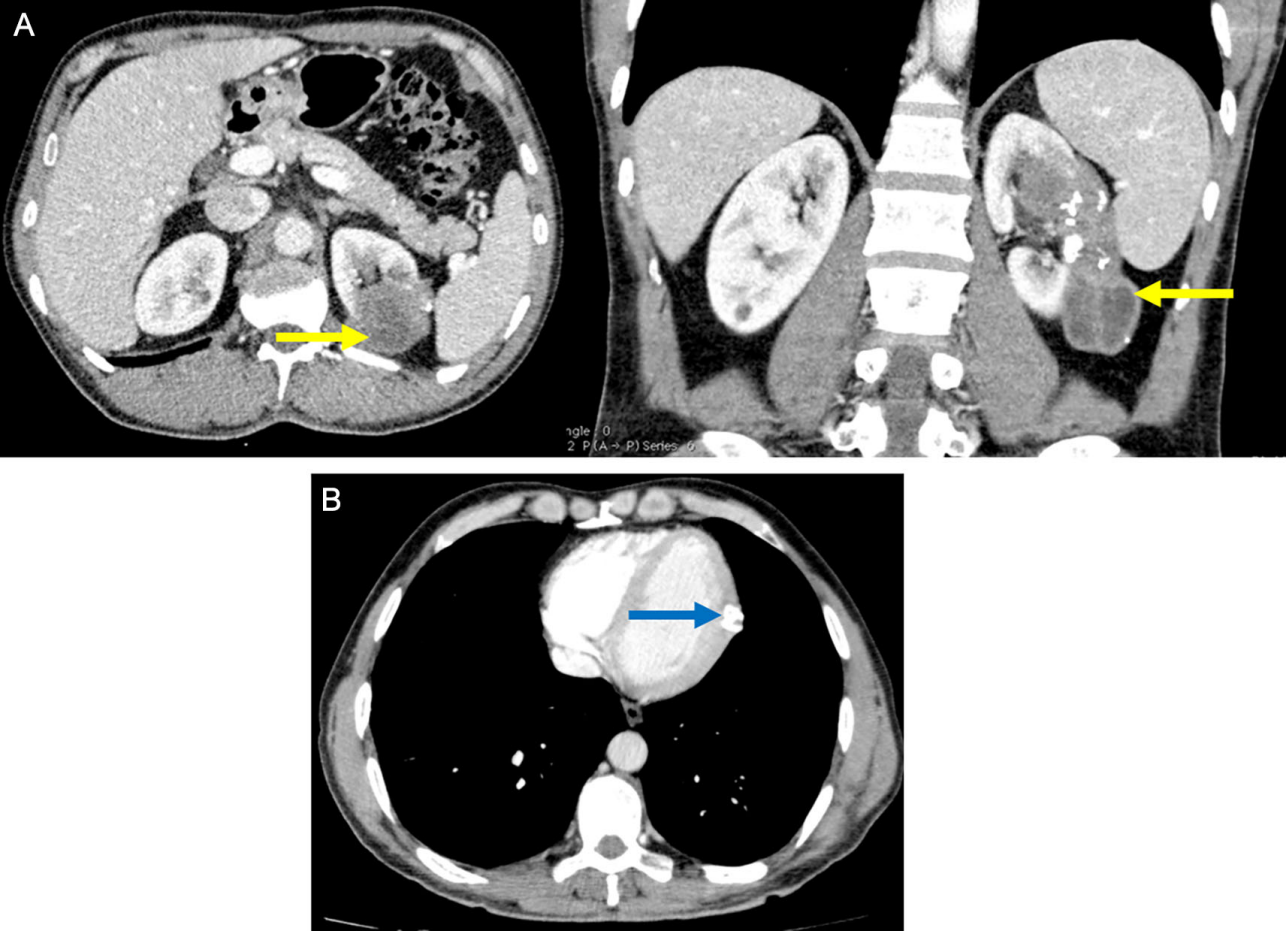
Axial computed tomography scans passing through the thorax in the mediastinal window and through the kidneys and coronal through the kidneys after injection of iodinated contrast product: Calcified hydatid cyst of the lateral wall of the left ventricle (blue arrow). Partially calcified multivesicular hydatid cyst with left endo and exo renal development (yellow arrow).
